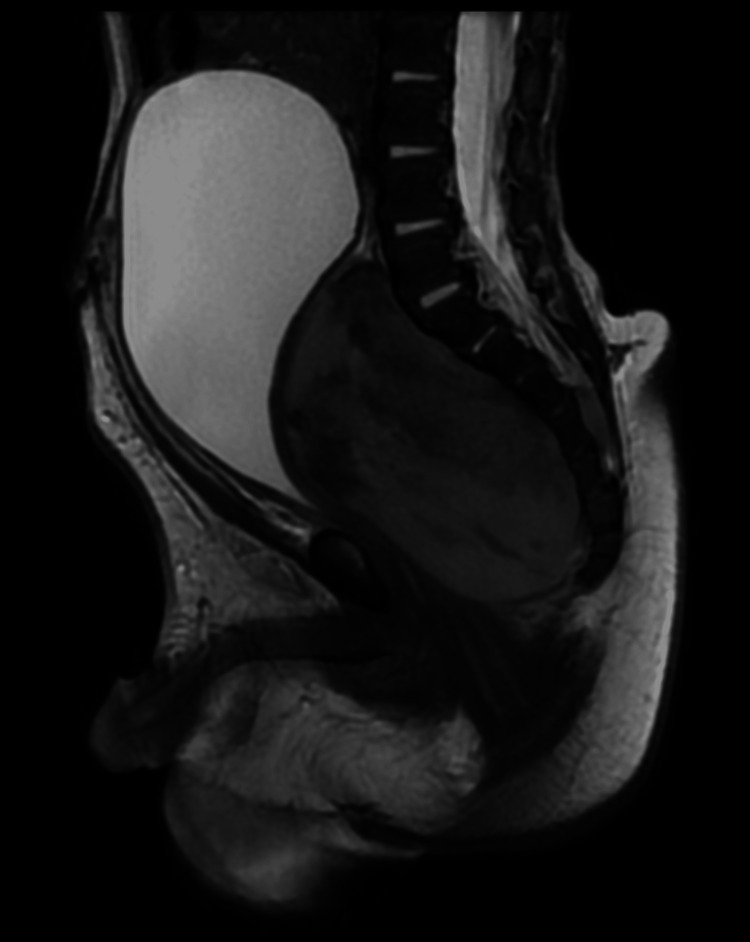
A sagittal image of the MRI scan revealed a large heterogeneous presacral mass extending to the spinal canal through the exiting neural foramina.
